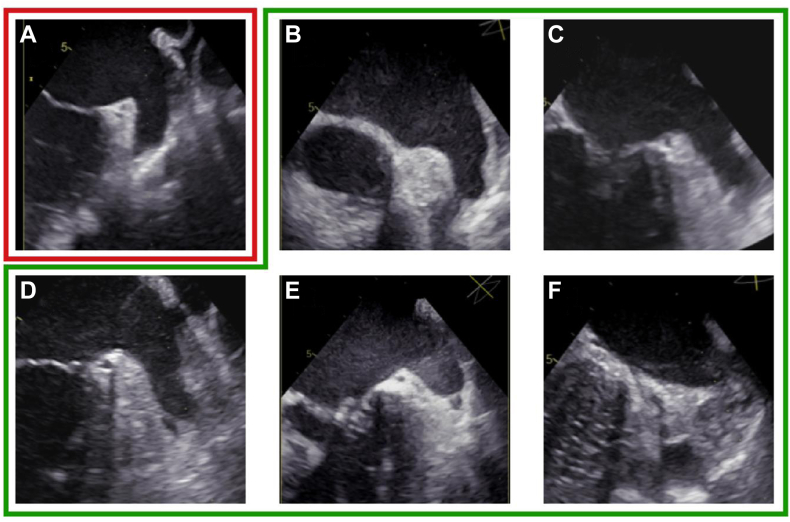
LATS stages. A: SEC 1+ : minimal echogenicity, typically confined to the LAA. B: SEC 2+ : mild to moderate swirling, detectable without increased gain. C: SEC 3+ : moderate, dense swirling, persistent throughout the cardiac cycle. D: SEC 4+ : severe echodensity with slow, layered swirling extending into the main LA cavity. E: LAA sludge: dense, gelatinous echodensity without a discrete mass, suggestive of pre-thrombotic state F: LAA thrombus: organized, well-defined mass. TOE = transesophageal echocardiography; LATS = left atrial thromboembolic substrate; SEC = spontaneous echo contrast.
